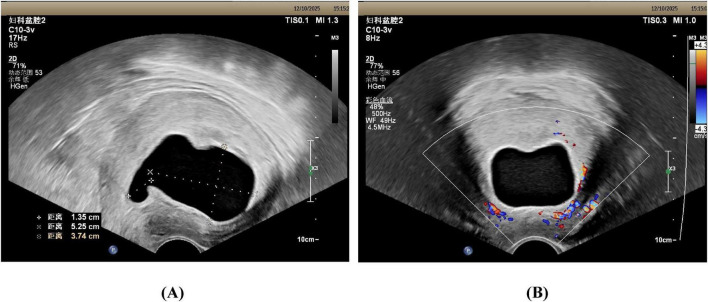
Preoperative transvaginal ultrasonographic findings. (A) B-mode ultrasound demonstrating a large, unilocular, anechoic cystic lesion (approximately 65 mm × 45 mm) with a thickened wall, occupying the uterine cavity and initially suspected to represent an empty gestational sac. (B) Color Doppler imaging revealing minimal peripheral vascularity surrounding the lesion, with no significant internal blood flow detected.
